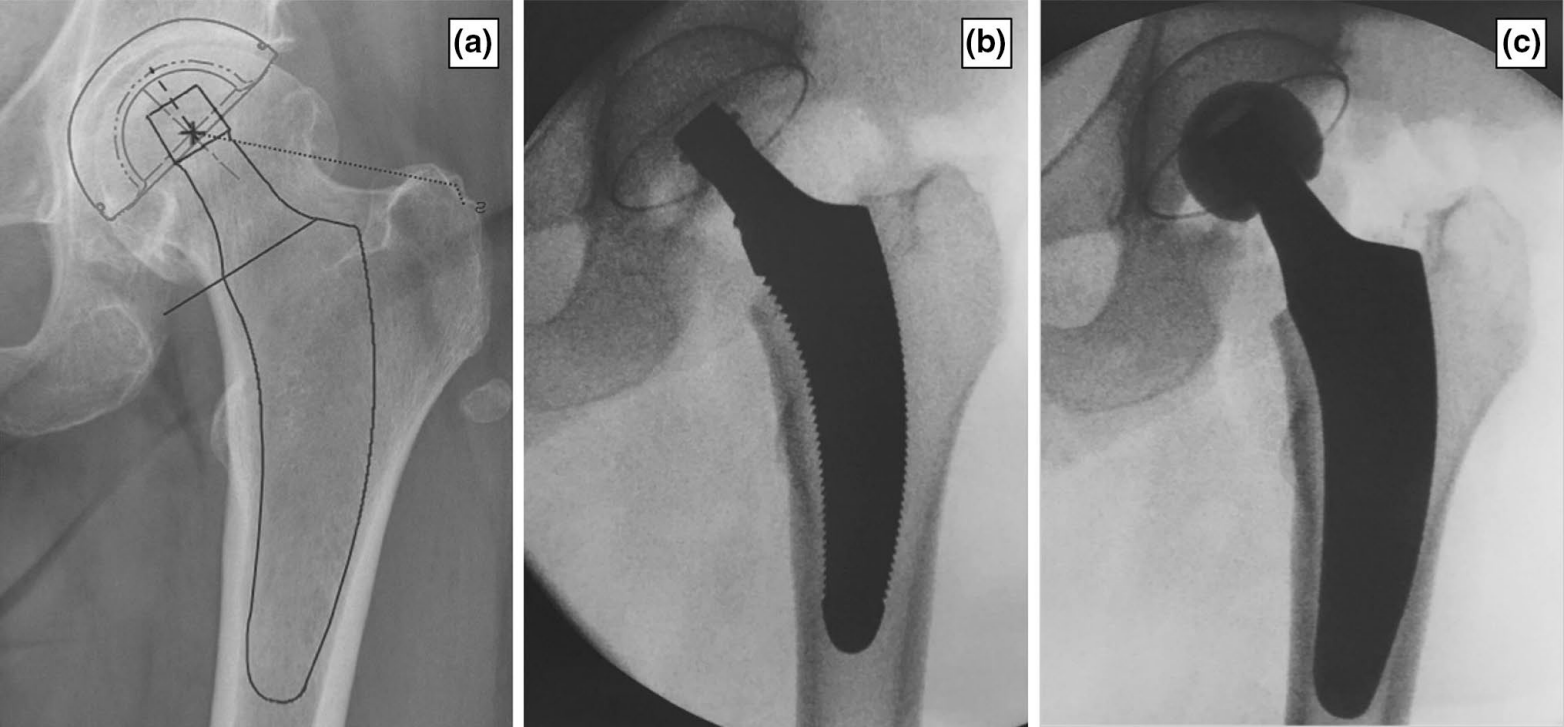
a Preoperative planning. b Intraoperative radiography with the trial implant of the planned size. Compared to the preoperative planning, it appears to be undersized and misses lateral cortical contact. c Radiography with the original implant after upsizing. The result shows adequate positioning matching the preoperative planning
